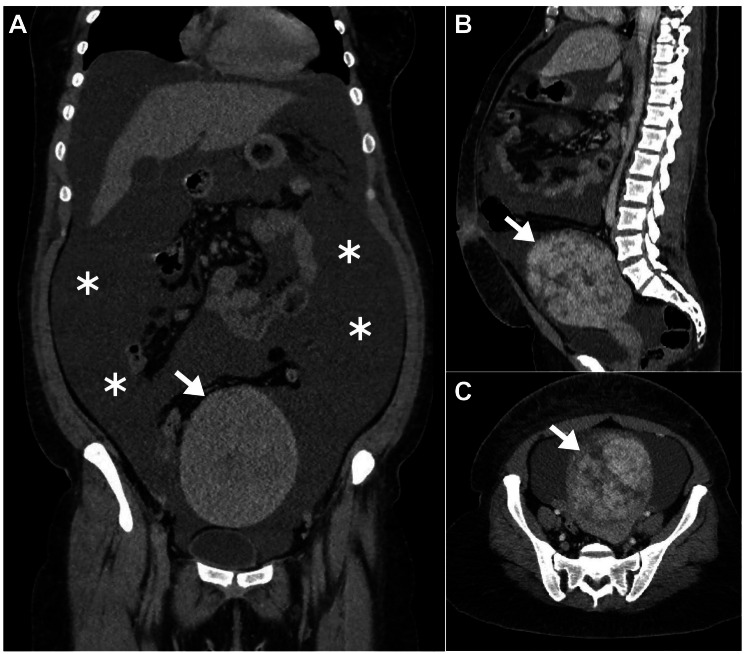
Abdominopelvic CT. (A) Coronal abdominal CT revealed massive ascites (asterisks) with evidence of a large uterine mass budding toward the abdominal cavity (arrow). (B) Sagittal CT and (C) axial CT revealed evidence of massive uterine enlargement with heterogeneous density (arrows). CT: computed tomography
